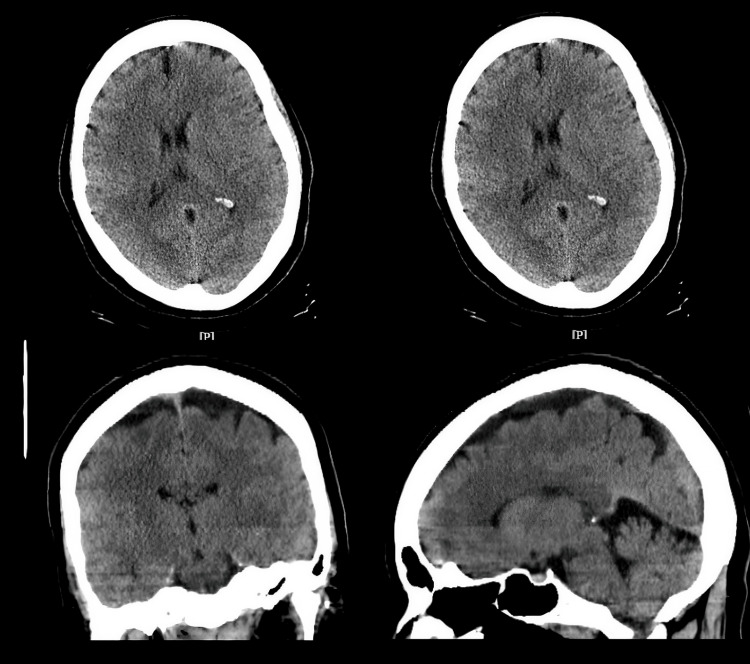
Non-contrast computed tomography (CT) of the brain. There was no evidence of acute intracranial hemorrhage, mass effect, or midline shift. Ventricular size and cortical sulci appear within normal limits for the patient's age, and no focal parenchymal abnormalities were identified.
